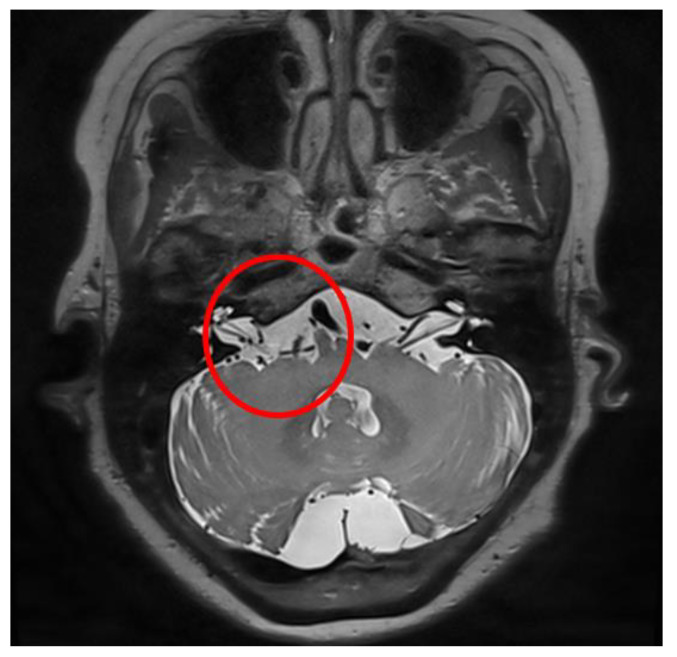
A 59-year-old woman with right HFS. Preoperative proton density-weighted (PD-weighted) magnetic resonance imaging (MRI). In the exit zone of the right facial nerve root, the right vertebral artery (VA) and anterior inferior cerebellar artery (AICA) were compressed in a tandem pattern (red circle).
